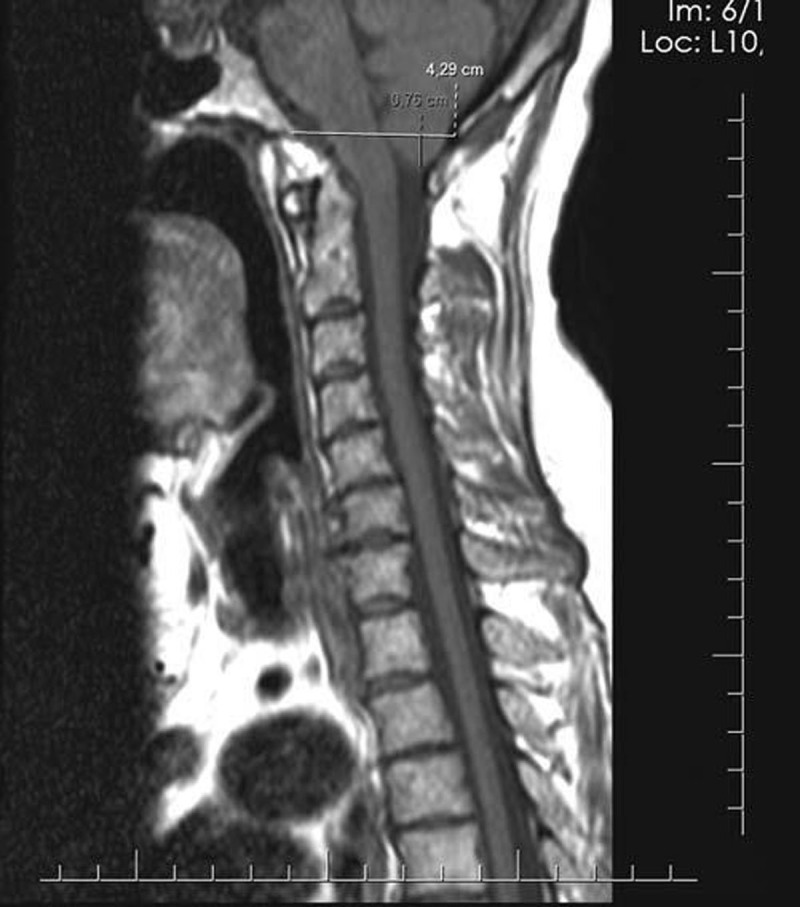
Sagittal T1-weighted TSE image showing cerebellar tonsils below the level of the foramen magnum.
